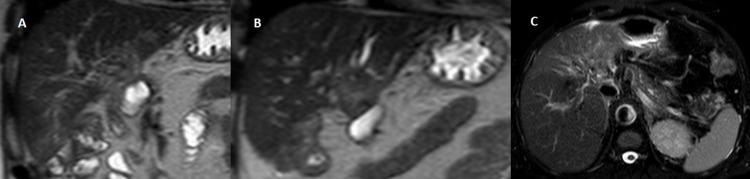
MRI shows a slightly hyperintense T2w irregular lesion in lateral segments of left lobe, with dilatation of the intrahepatic bile ducts ({A and B} coronal T2w SSh, {C} axial T2w FSE fat sat). T2w SSh: T2-weighted single shot; T2w FSE fat sat: T2-weighted fast spin-echo fat-saturated
